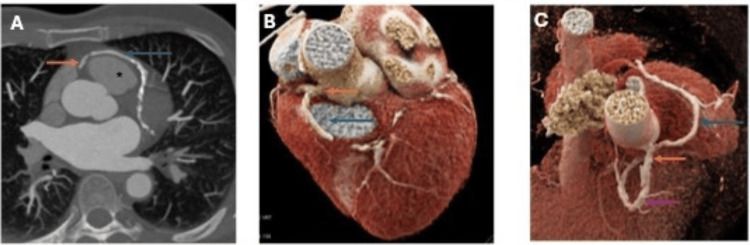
(A) Axial thick sections showing the left main coronary artery (blue arrow) arising from the right coronary sinus (orange arrow) and passing anterior to the pulmonary artery (*). (B and C) Volume-rendered CT images with the left main artery (blue arrow) arising from the right coronary sinus (orange arrow). Distal right coronary artery is also seen (purple arrow).
